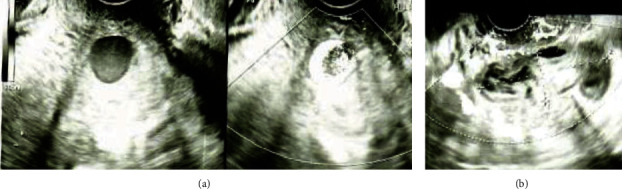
Transvaginal ultrasonographic images. (a) The left image shows the B-mode scan, and the right image shows color Doppler scan. To the left of the uterus, a 1.7 × 1.6 cm low-echoic mass is observed with turbulent flow inside it. (b) An 8 cm sized hematoma is observed in the pelvis.
